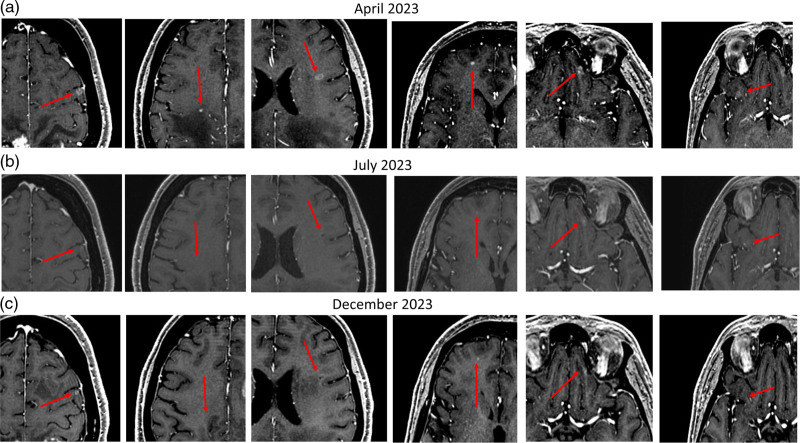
Brain MRI, axial sections of T1-weighted images after gadolinium administration. (a) during treatment with pralsetinib at a reduced dose, progression of the disease was documented by the appearance of multiple brain metastases (red arrows) in different cerebral lobes characterized by both cortical-subcortical and deep distribution, small size without significant vasogenic edema. (b) three months after starting selpercatinib, partial response was proved by the reduction of the size of the multiple cerebral metastases; some lesions disappeared such as the lesion of the deep white matter of the right frontal lobe and the cortical-subcortical lesion of the left inferior frontal gyrus. (c) six months after starting selpercatinib, the persistent cerebral response was maintained for all the cerebral lesions.
